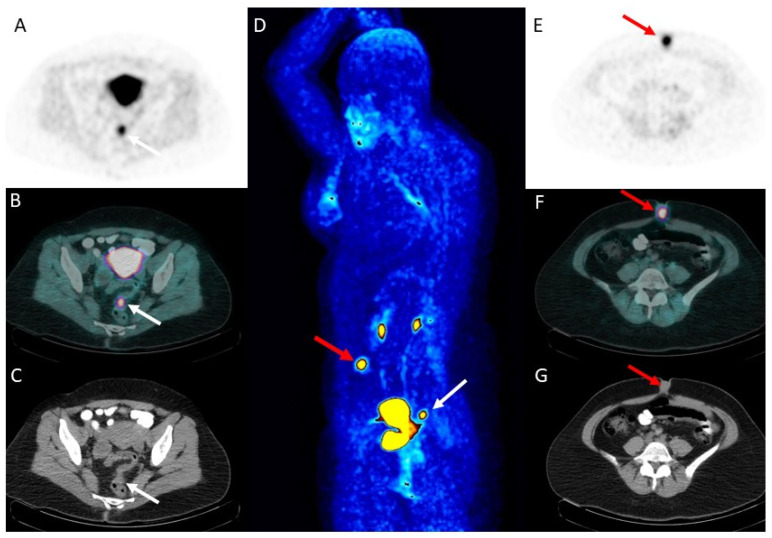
A 38-year-old female underwent [^68^Ga]Ga-FAPI-04 PET/CT due to suspected endometriosis. [^68^Ga]Ga-FAPI-04 PET/CT [(A,E): emission trans-axial PET images; (B,F): trans-axial fused PET/CT images; (C,G): trans-axial CT images; (D): maximum intensity projection—MIP] shows physiological radiotracer accumulation in the kidneys and bladder due to renal excretion and in correspondence with the uterus. Moreover, two areas of focal radiotracer uptake are detected in the rectum/sigmoid colon (white arrows) and in the umbilical area (red arrows). Following [^68^Ga]Ga-FAPI-04 PET/CT, the lesion detected at the umbilical area was biopsied and confirmed as a focus of endometriosis.
